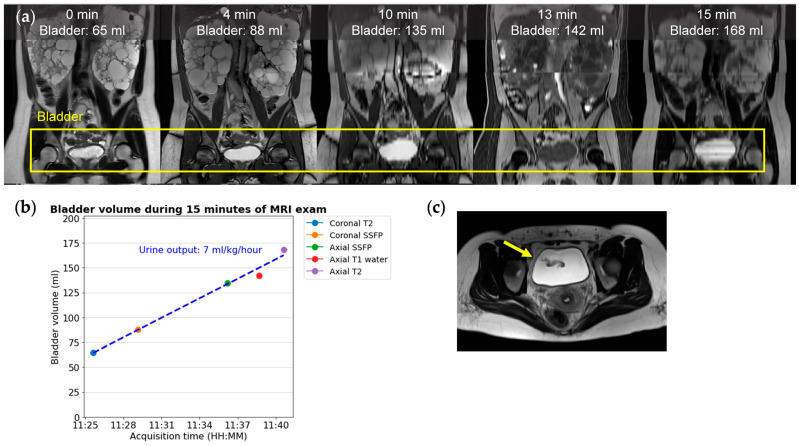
In this 36-year-old ADPKD female patient, the urine output is 7 mL/kg/hour, estimated from serially acquired images during a 15 min MRI exam of 5 sequences; (a) from left to right: coronal T2, coronal SSFP, coronal reformation of axial SSFP, T1, and T2. (b) A graph of urine output corresponding to the slope of a linear regression fit to the bladder volume divided by the subject’s weight. The DICOM image timestamp is treated as the instantaneous acquisition time for each image. In our experience, T1 (red dot) commonly shows a lower bladder volume compared to other sequences. (c) An axial T2 image with a ureteral jet in-flow signal void artifact (yellow arrow).
